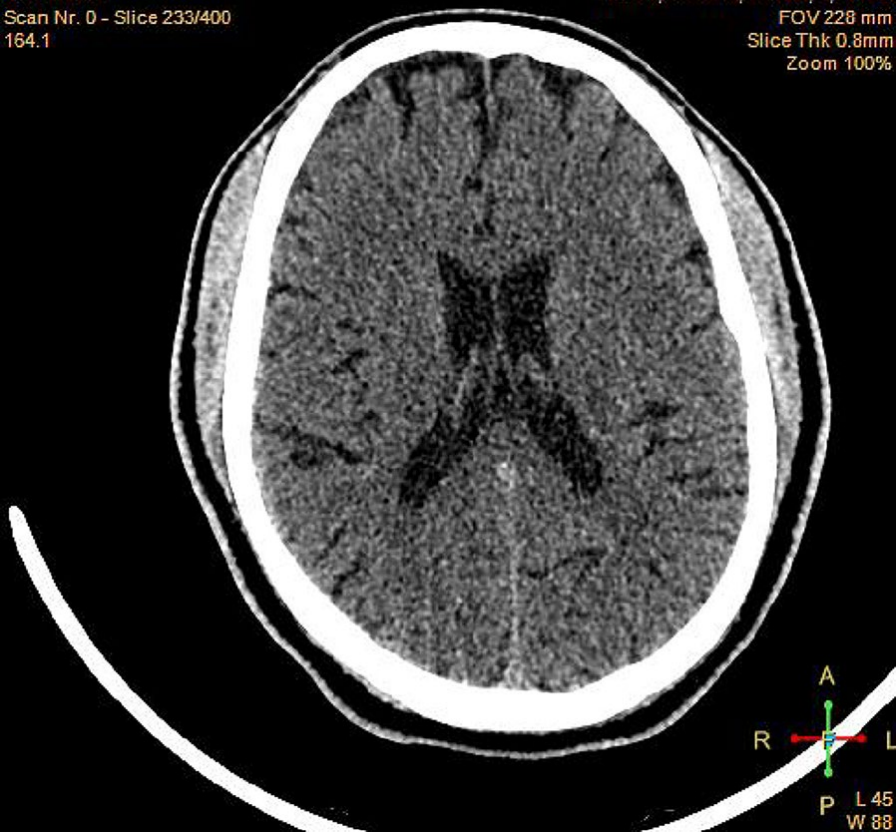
Shows normal CT-scan of the patient presented with right upper limb hemichorea due to hyperglycemia in type D.M patient in Moshi Tanzania
